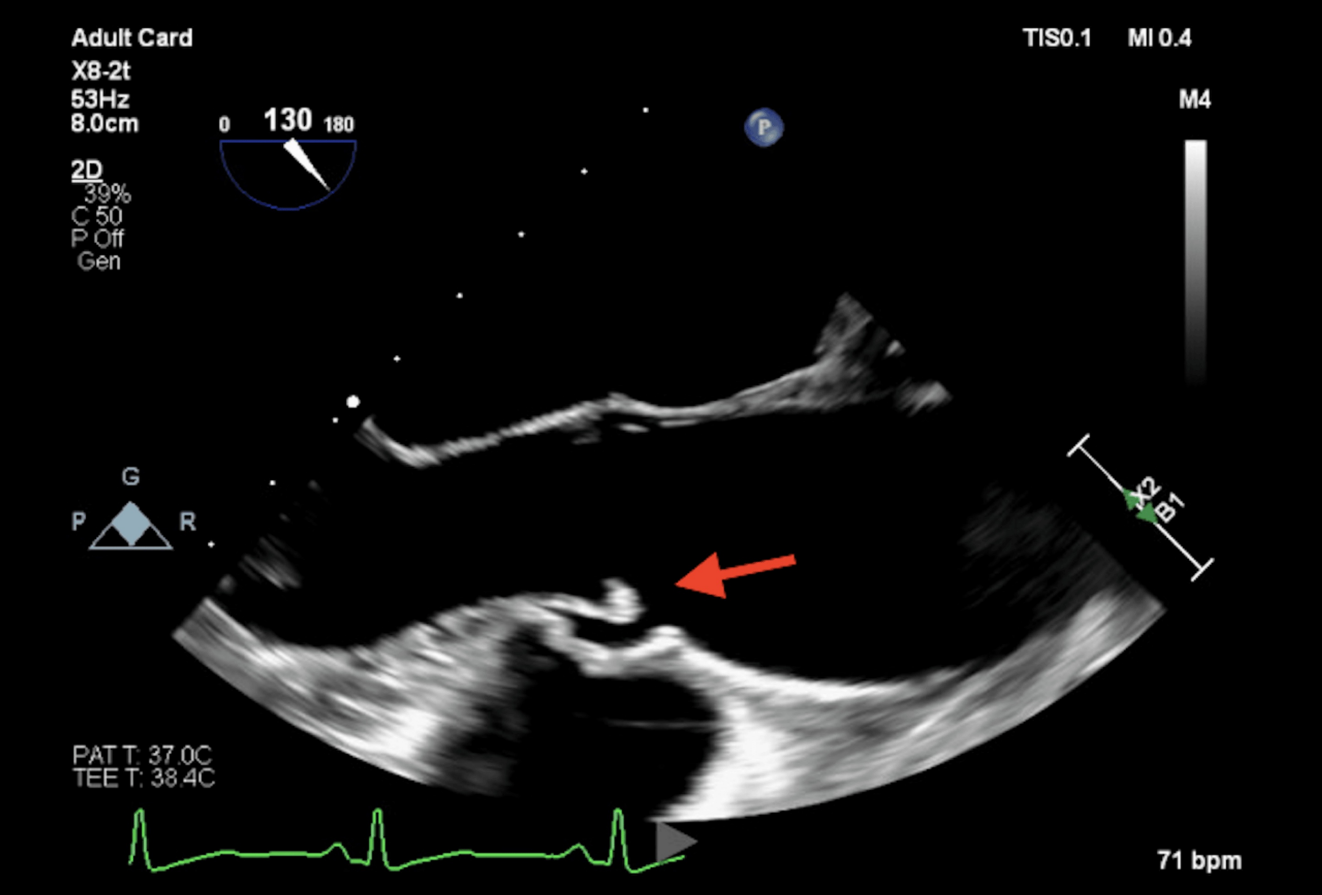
Nonbacterial thrombotic endocarditis on transesophageal echocardiography. Transesophageal echocardiography findings in the midesophageal aortic valve long axis view with mild thickening and prominent focal thickening of the tip of the right coronary cusp (red arrow) were suggestive of nonbacterial thrombotic endocarditis.
